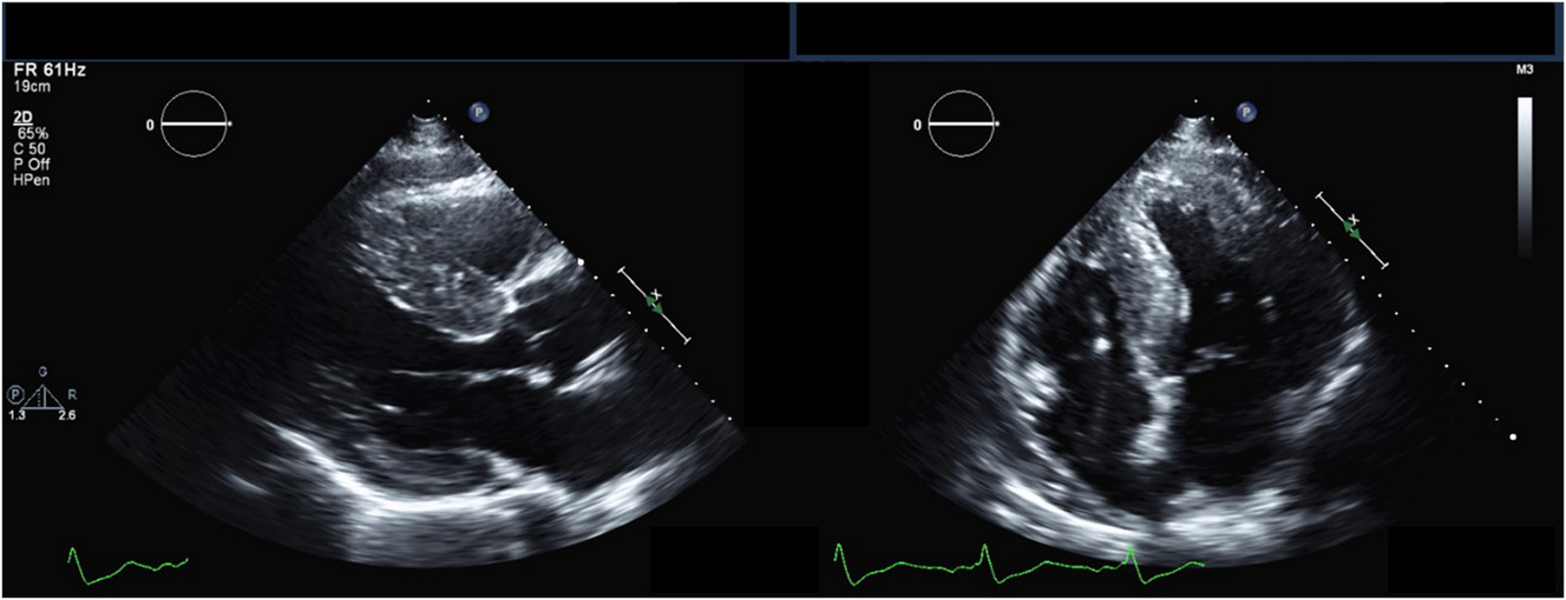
Echocardiography findings in Anderson-Fabry disease. Parasternal long-axis (left) and apical 4-chamber (right) transthoracic echocardiography views demonstrating increased left ventricular wall thickness.
